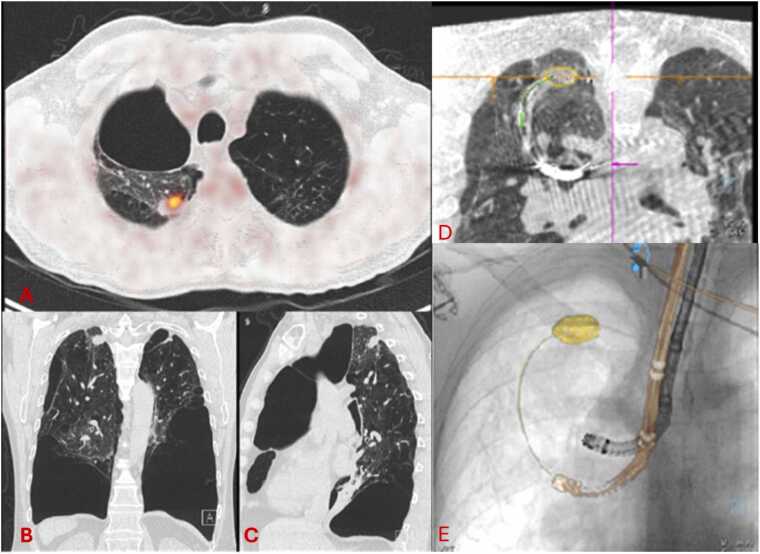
A; PET-CT scan of patient 2 in axial plane with FDG-avid hotspot in right upper lobe. B and C; CT scan of patient 2 in coronal and sagittal plane with nodule in right upper lobe with pronounced bullous emphysema. D: Cone beam CT image with colored lesion in right upper lobe (Yellow) and endobronchial route (Green) E: overlay augmented fluoroscopy during bronchoscopy to reach nodule in right upper lobe.
